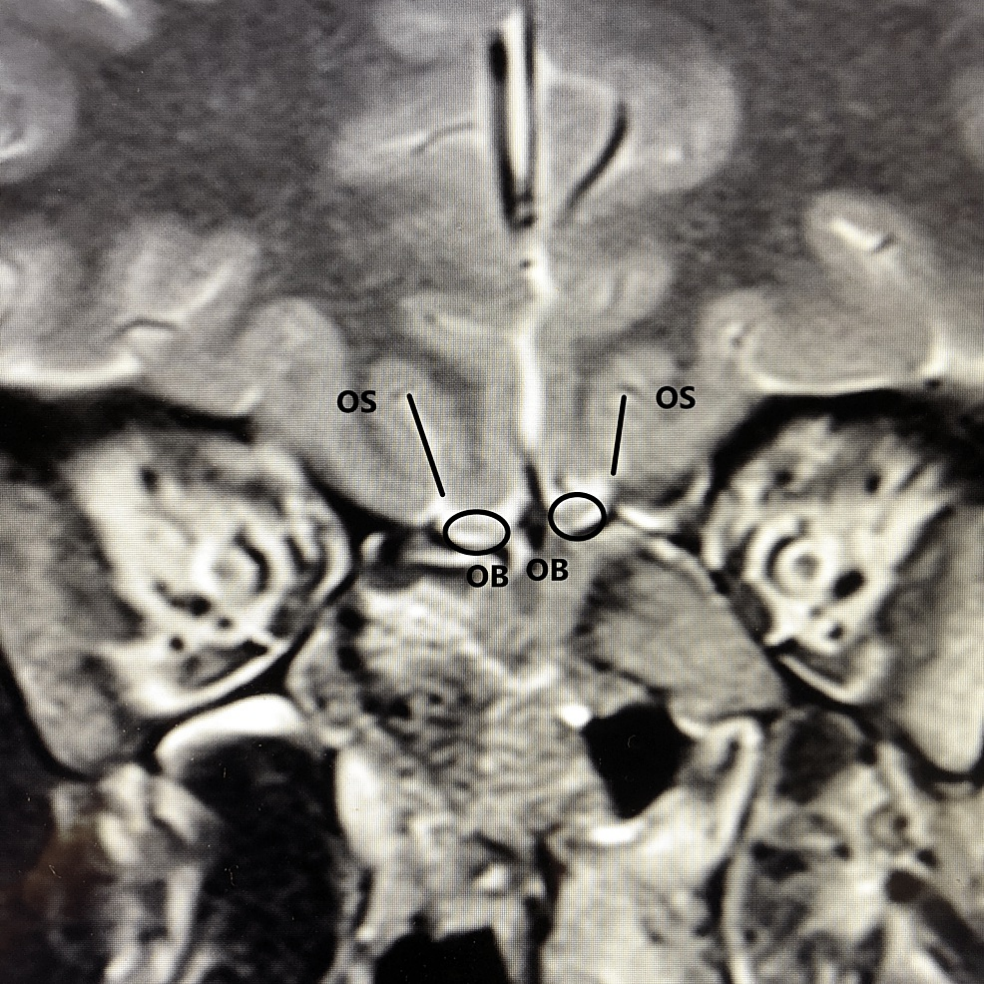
Coronal T2 MRI showing the olfactory bulb and olfactory sulcus.
